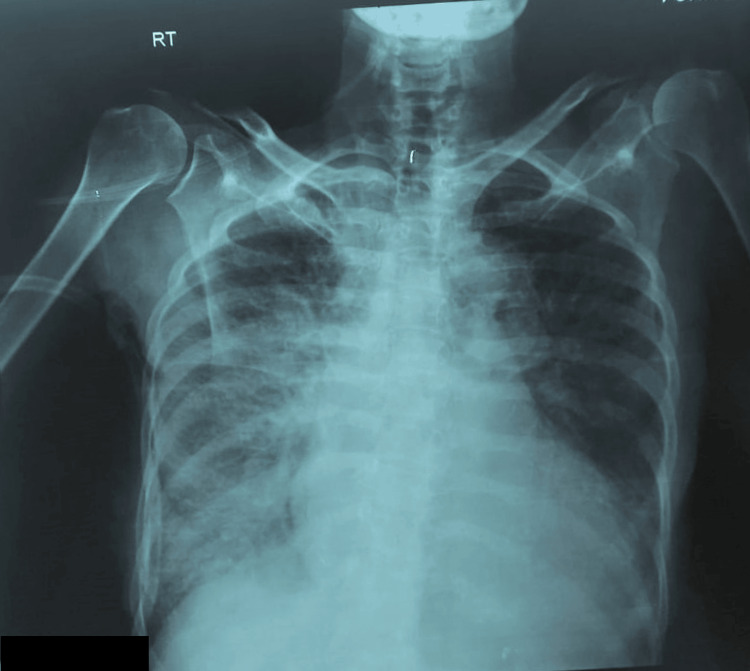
Chest X-ray showing pulmonary oedema and cardiomegaly
